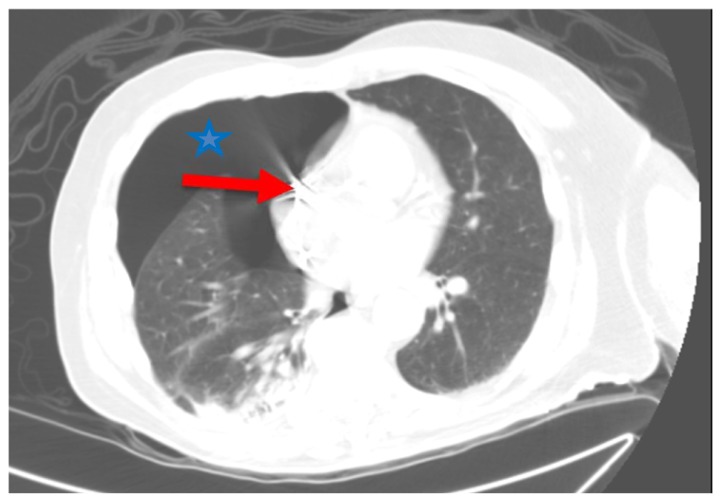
CT scan of the chest showing right-sided pneumothorax (blue star) and the tip of pacemaker lead perforating the myocardium (red arrow).
